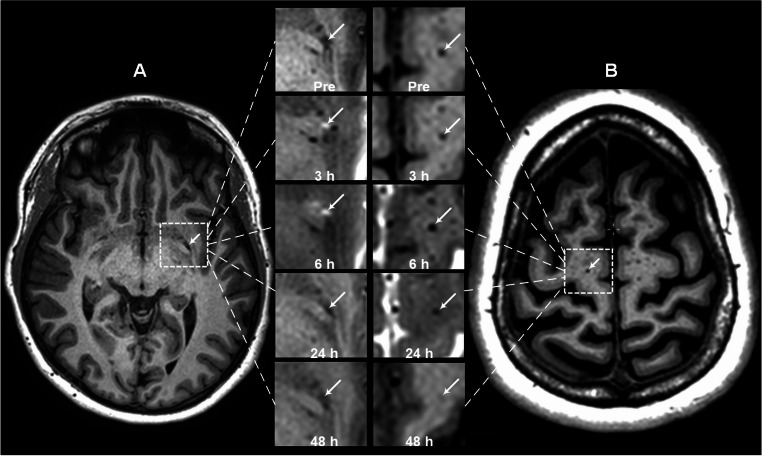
MRI-visible perivascular spaces (PVS).T1-weighted MRI zoomed in on a section (stippled square) of basal ganglia (upper row) and subcortical white matter (lower row). An MRI-visible PVS in each region is pointed out with arrows. A circular region of interest was manually adapted within each PVS at each time point, allowing for assessment of percentage T1 signal increase, where increased T1 signal indicates increased tracer enrichment.
